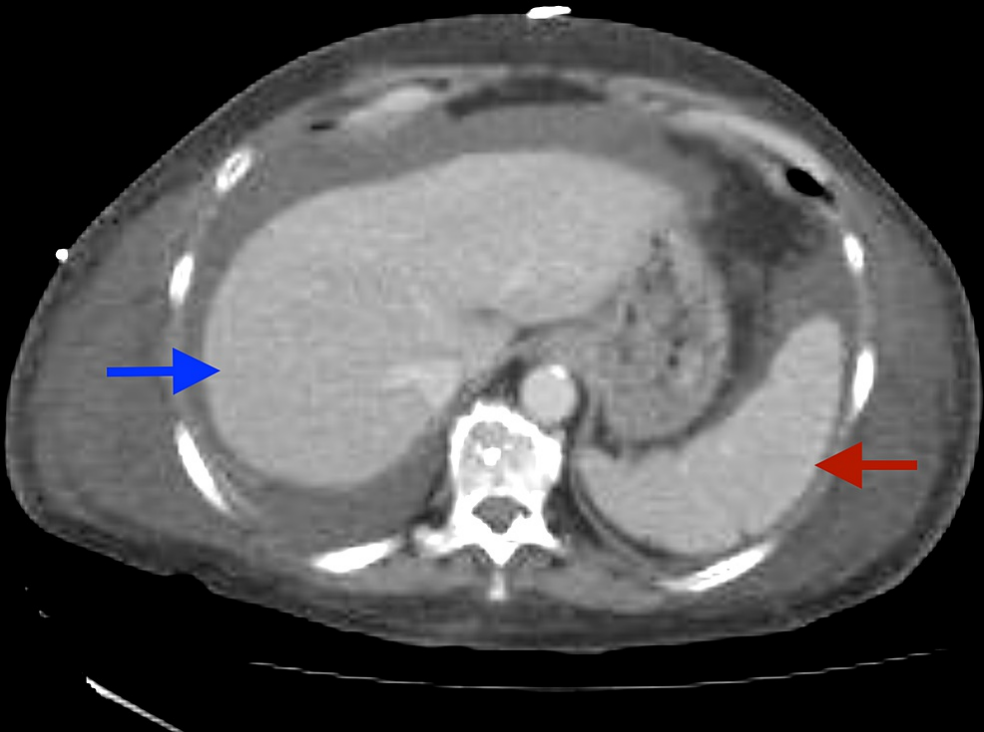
CT abdomen CT of the chest, abdomen, and pelvis performed after Cycle 1 of CHOP (cyclophosphamide, doxorubicin hydrochloride, vincristine sulfate, and prednisone) showed anasarca with ascites and pleural effusion. Notably, as seen on this representative image, the liver (blue arrow) and spleen (red arrow) appeared normal with no evidence of hepatosplenomegaly or of any extramedullary involvement of T-cell lymphoma.
